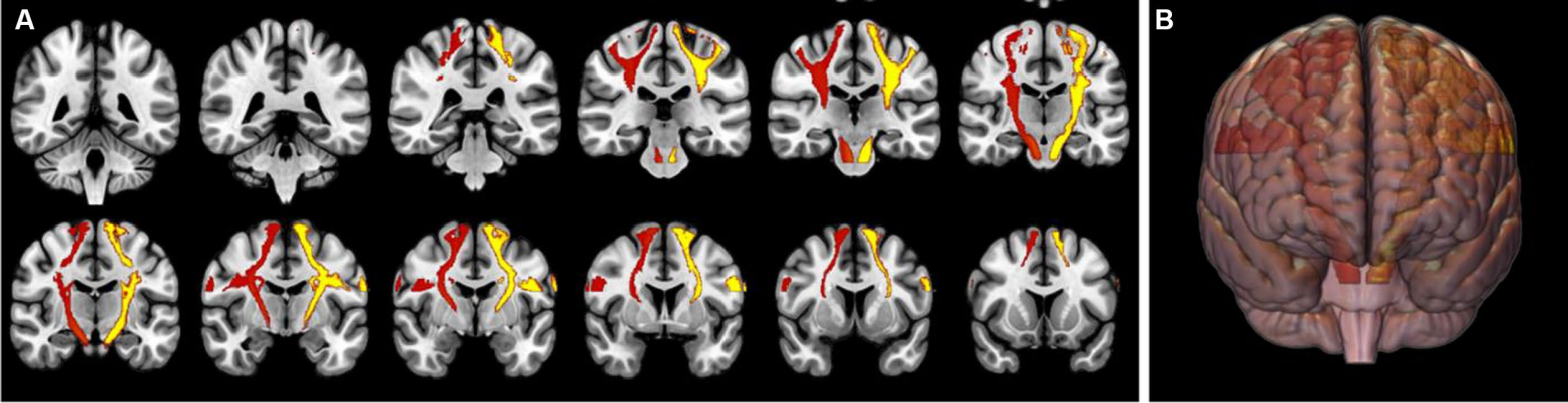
Volume of interests of both of the corticospinal tracts (in red and yellow) at a distance between the precentral gyri and the cerebral peduncles overlaid on a 2D coronal structural T1-weighted image (A) and a 3D brain visualization (B).
